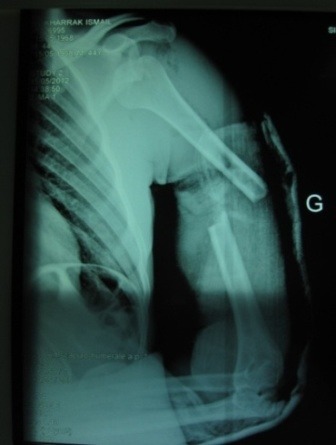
Radiographie de face de l’épaule montrant la luxation antérieure de l’épaule associée à une fracture de la diaphyse humérale homolatérale
